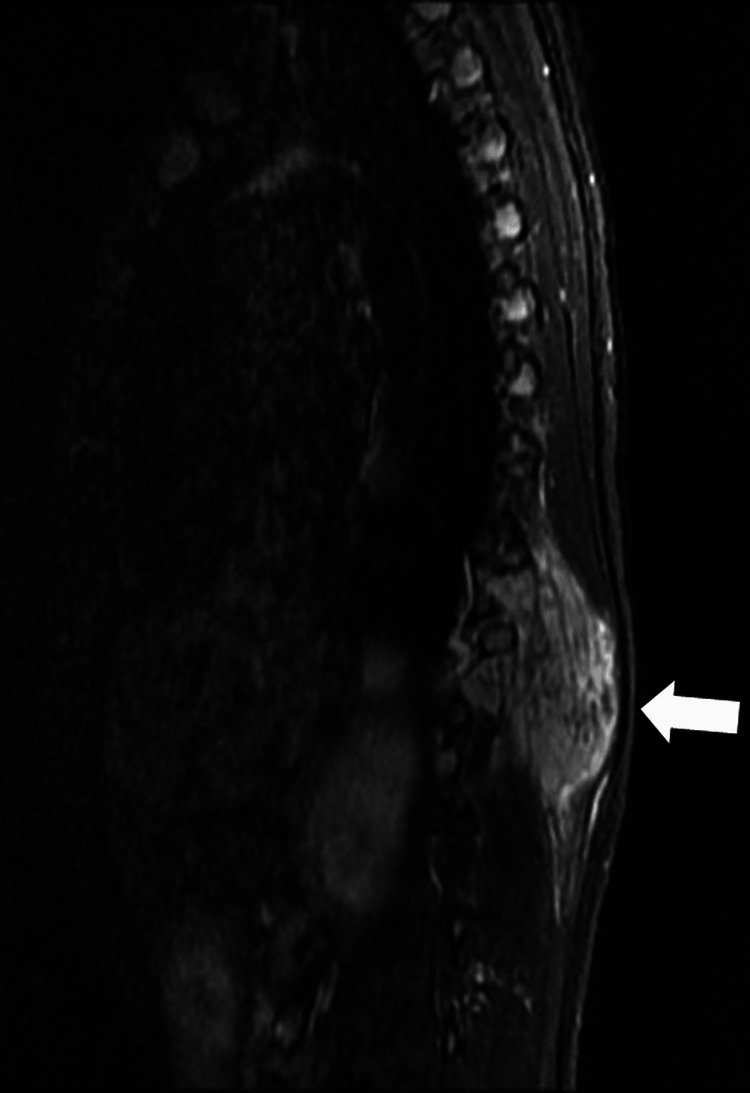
Saggital T1-weighted MRI post-contrast shows an intensely enhanced lesion (arrow).
